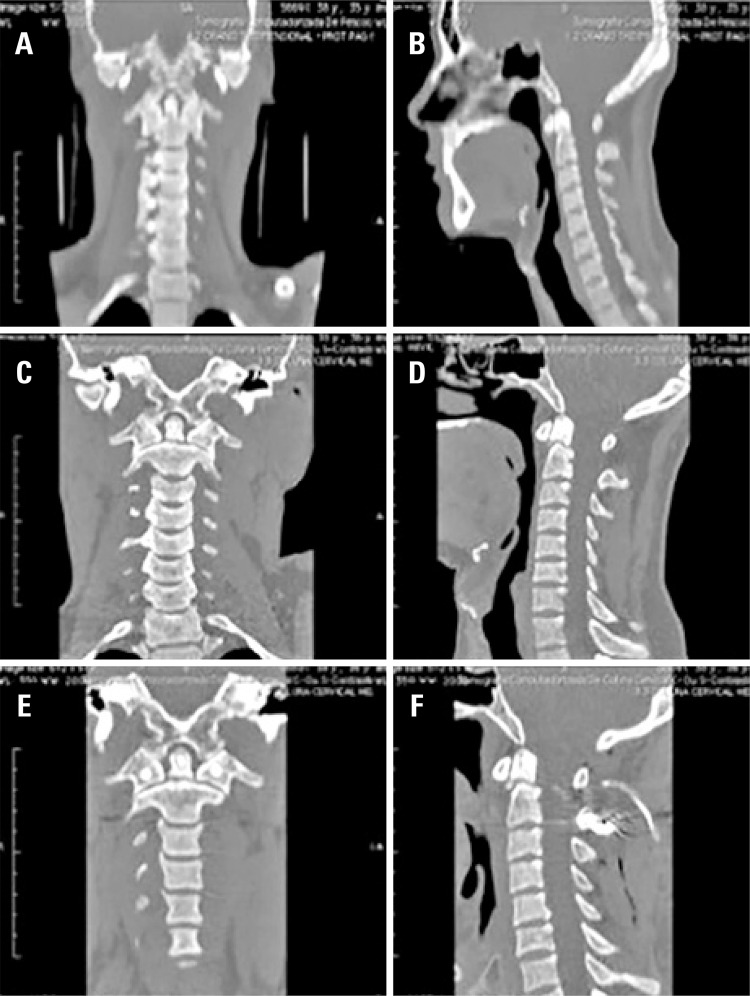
Odontoid fracture type II, conservative treatment failure. (A and B) non-comminuted odontoid fracture without deviation in a 37 years old patient, (C and D) computed tomography scan after eight weeks of conservative treatment showing, (E and F) surgical result after posterior C1-C2 arthrodesis
